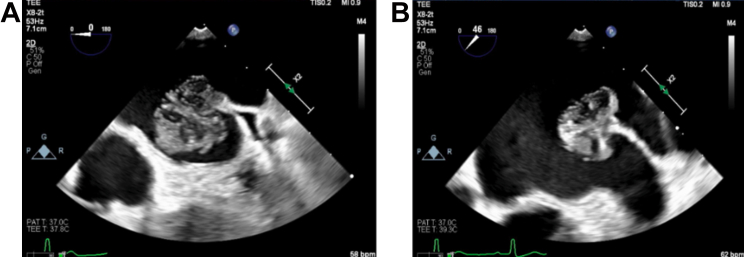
Trans-esophageal Echocardiogram Showing a Well-Circumscribed, Pedunculated Highly Mobile Mass With Irregular Frond Like Texture at the Coumadin Ridge Consistent With a Fibroelastoma (A and B) Transesophageal echocardiogram demonstrating the fibroelastoma on the Coumadin ridge.
